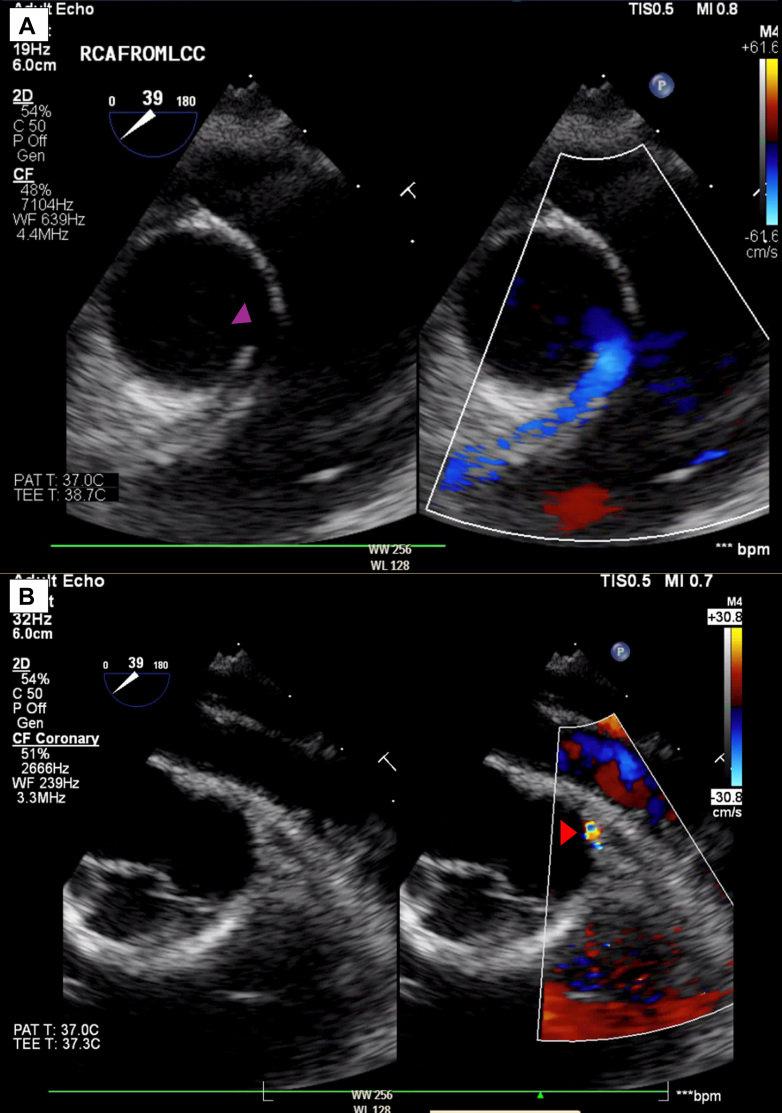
Initial Transesophageal Echocardiogram Transesophageal echocardiogram demonstrating (A) anomalous origin of the right coronary artery and (B) possible blind-ended coronary ostia at the typical left main coronary artery site.
